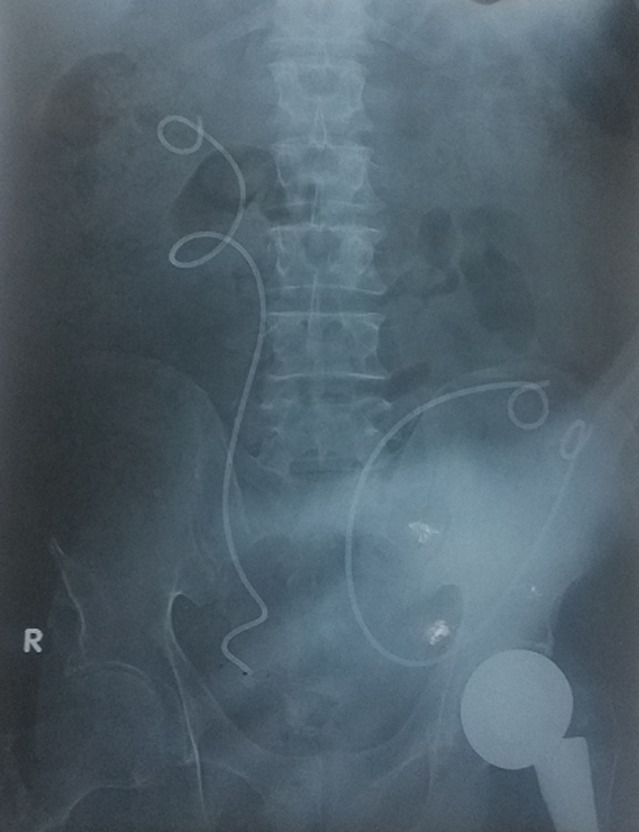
plain radiography X-ray showing both DJ stents after failed removal
